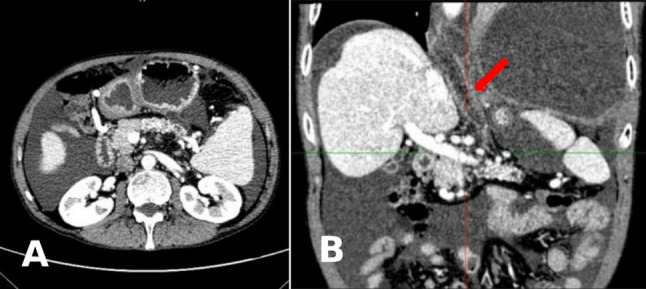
(A) Contrast-enhanced axial CT of the upper abdomen showing pancreatic atrophy with multiple clustered dense calcifications in the body and tail. (B) Coronal reconstruction image of contrast-enhanced upper abdominal CT demonstrating a tubular fluid-density tract (red arrow) extending upward from the pancreatic body through the esophageal hiatus toward the left pleural cavity
